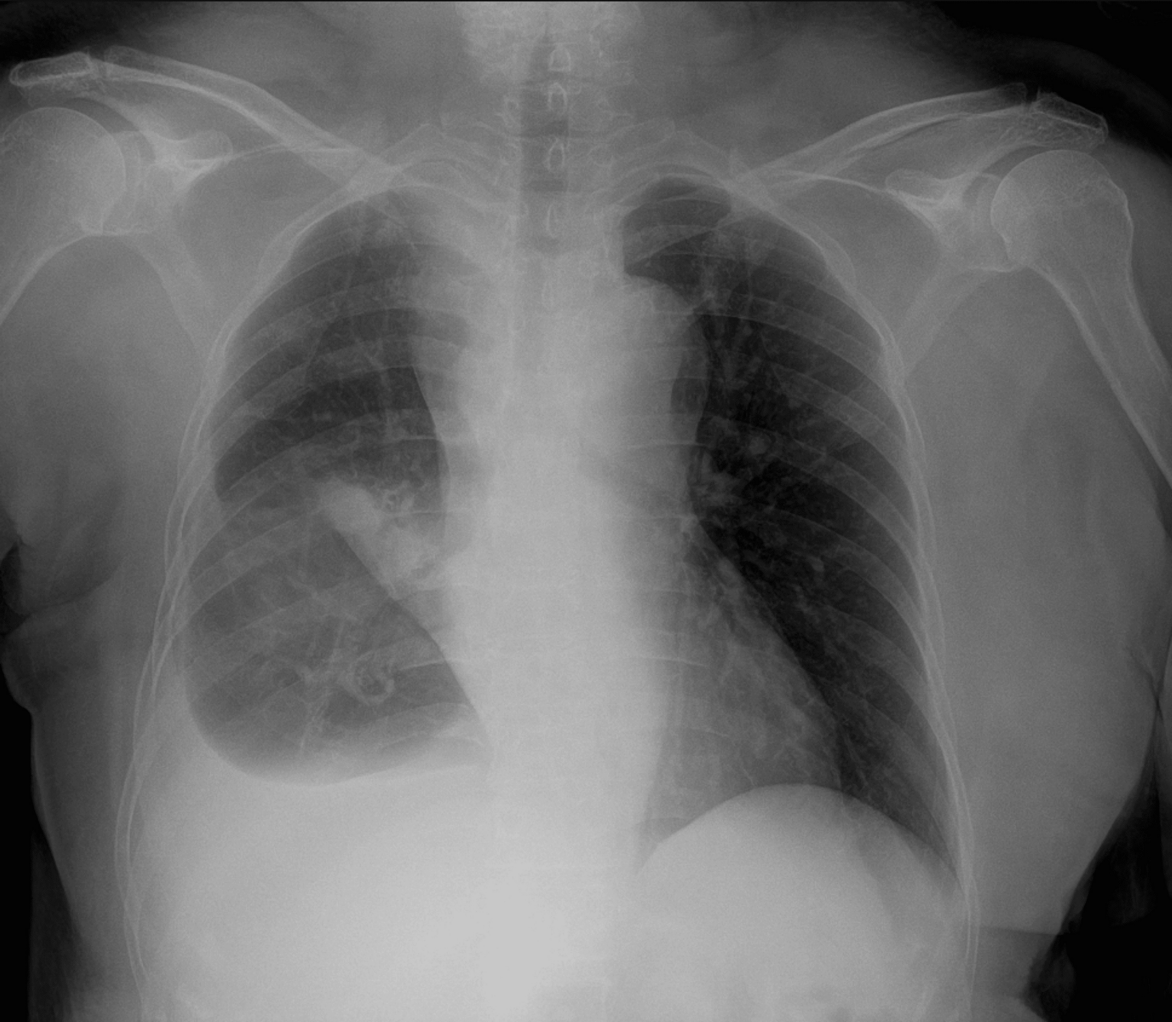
Pre-procedural frontal chest radiograph showing right pleural effusion.
